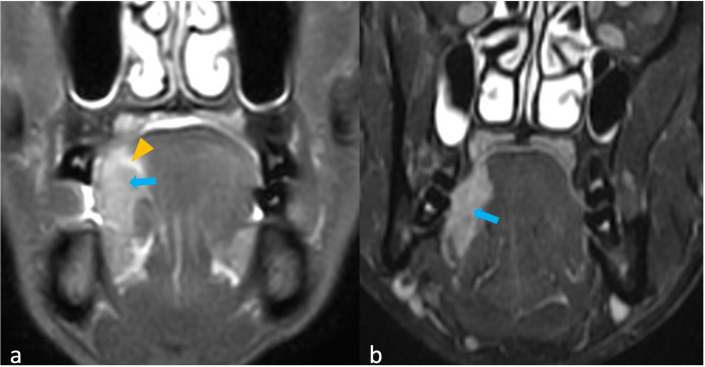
(a, b) MRI images of oral tongue squamous cell carcinomas with and without peritumoral edema (PE). (a) Coronal short tau inversion recovery (STIR) shows homogenously hyperintense PE (arrowhead) surrounding the heterogeneous right lateral border tumor (arrow). (b) Coronal STIR shows absence of PE surrounding the tumor (arrow).
